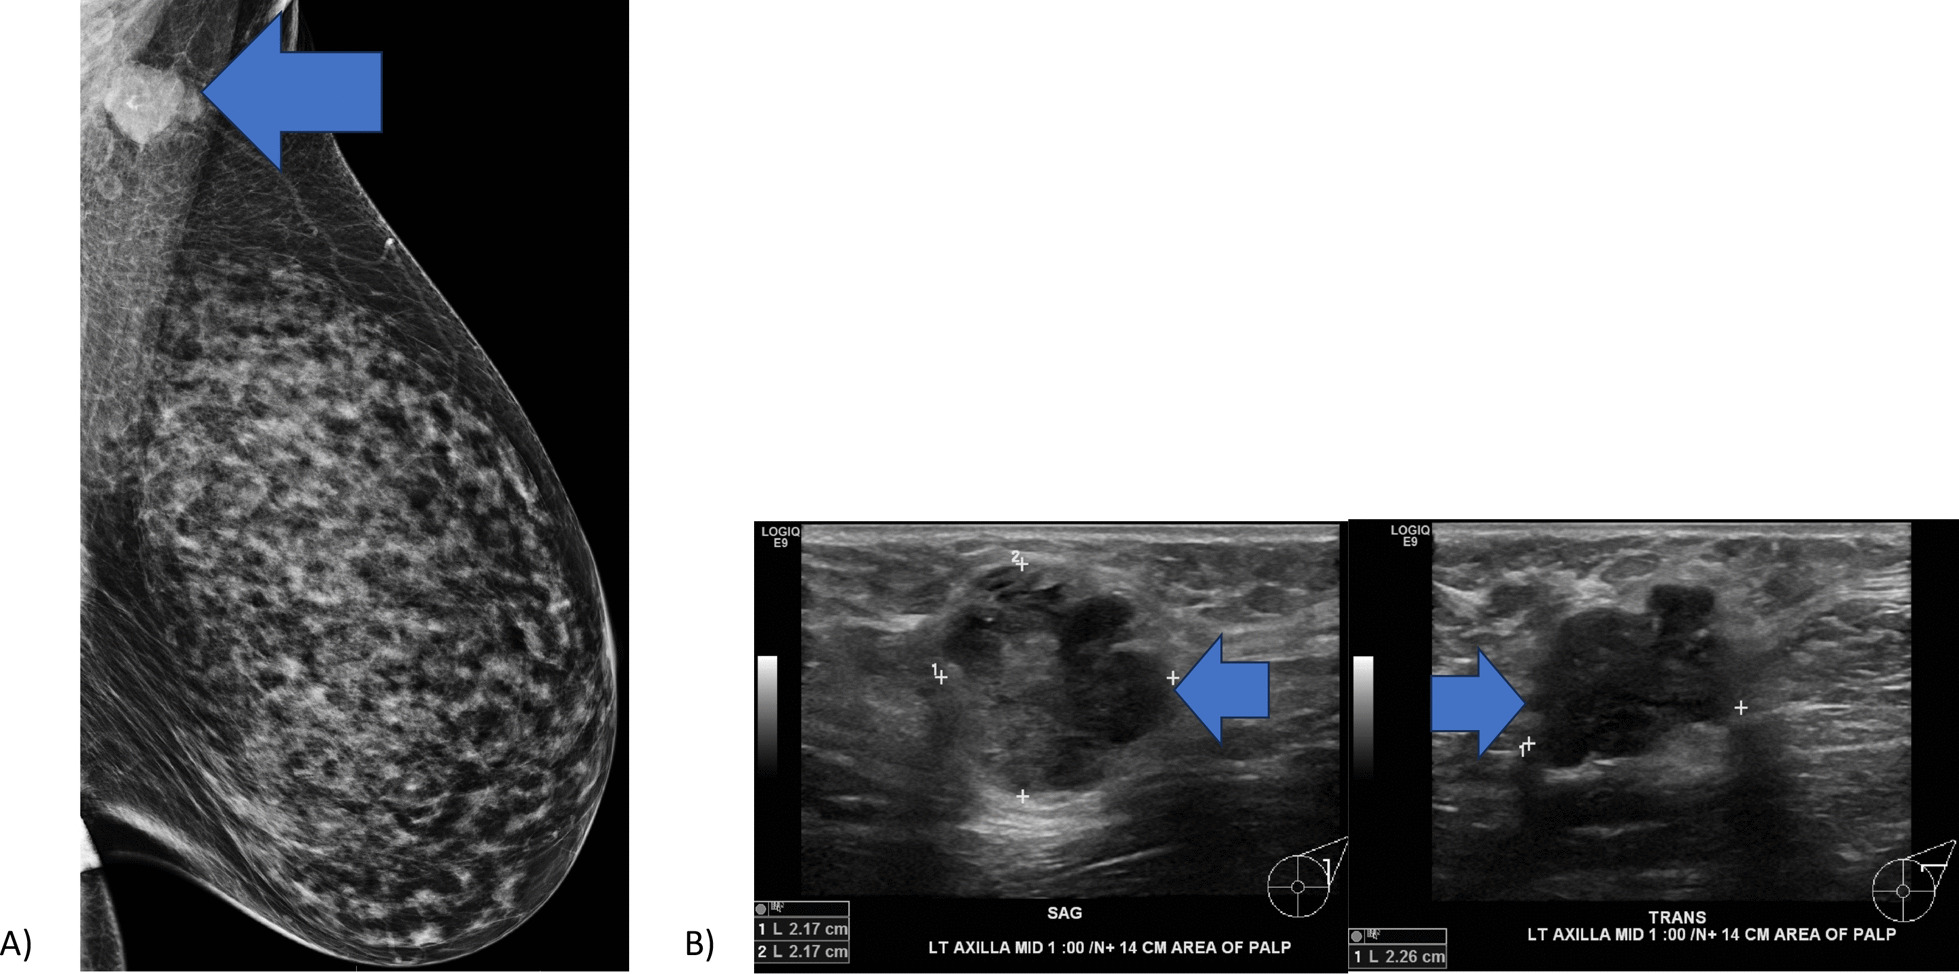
a Mammography of the left breast showing axillary LN enhancement (arrow) b Targeted ultrasound of the left breast showing hypoechoic regions in sagittal and transverse planes (arrows)
